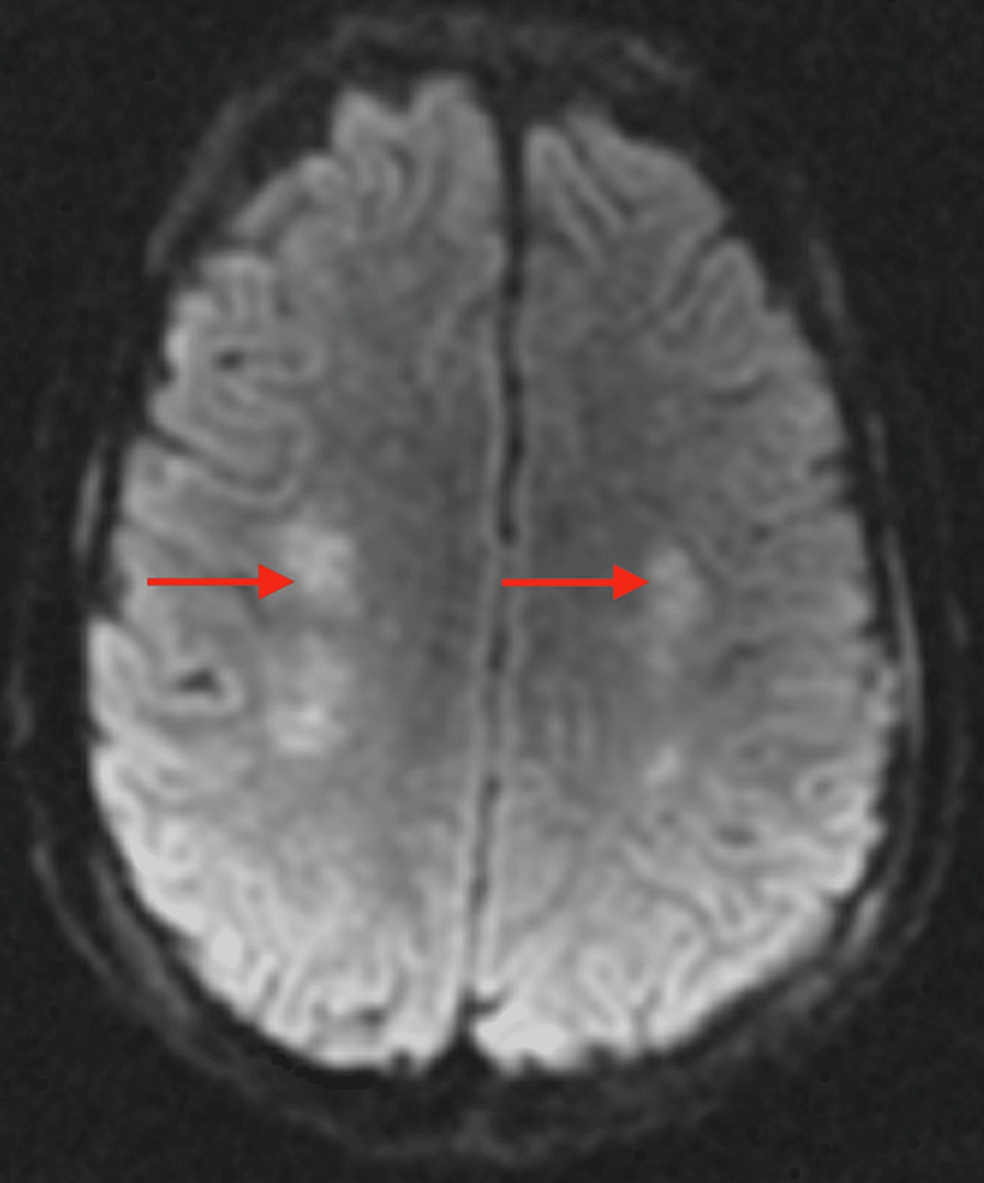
Axial DWI sequence of follow-up MRI showing symmetric bilateral diffusion restriction within the centrum semiovale (arrows)
